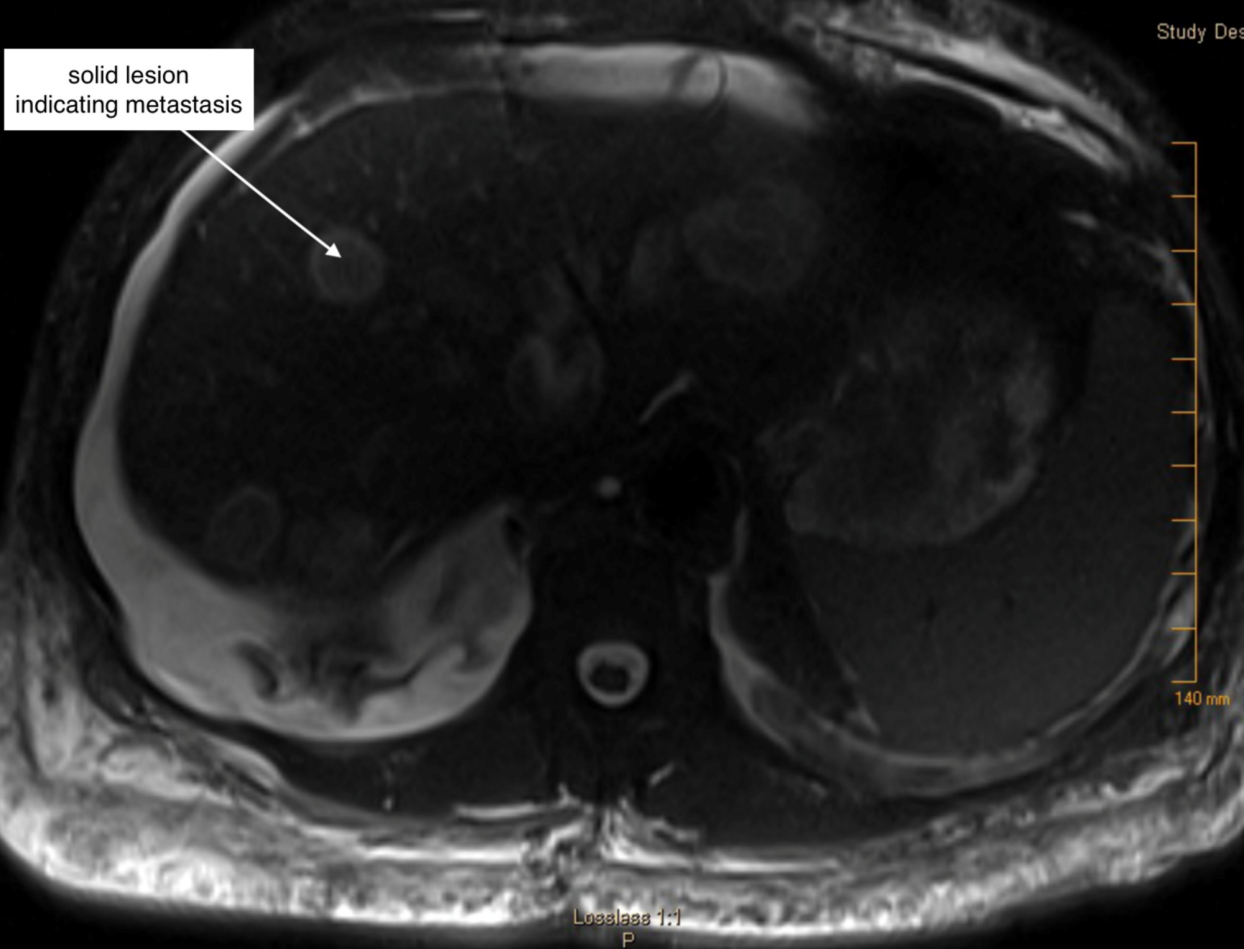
MRI abdomen showing multiple lesions in liver indicating metastasis
